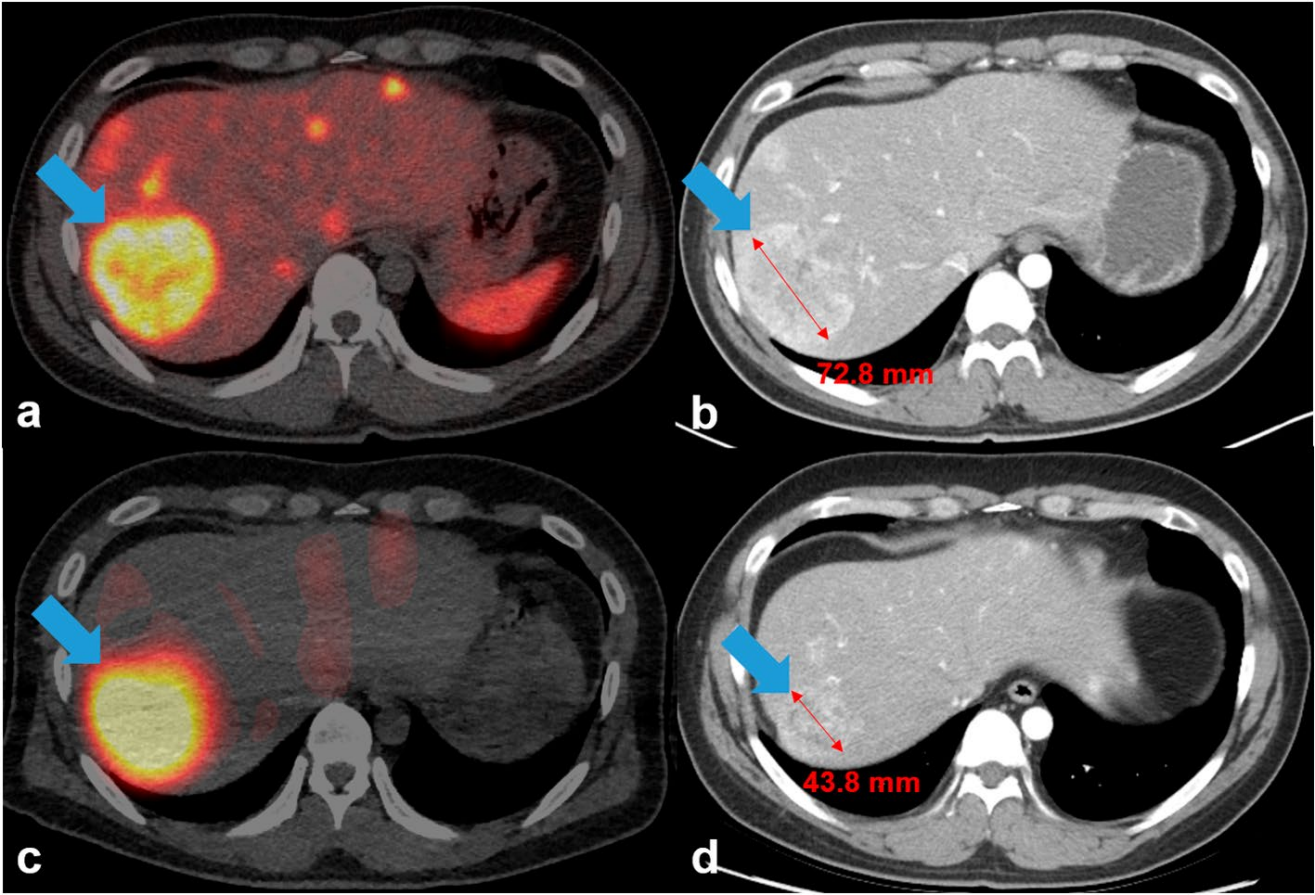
Representative images of the patient’s target lesion (liver) following [^177^Lu]Lu-DOTA-TATE therapy, showing a partial response (arrows). A Pre-therapeutic [^68^Ga]Ga-DOTA-TOC PET/CT exhibited a target lesion uptake in the liver that was more intense than normal liver uptake (Krenning score 3). B The lesion’s largest diameter measured 72.8 mm on pre-therapeutic CECT. After four cycles of [^177^Lu]Lu-DOTA-TATE therapy, C [^177^Lu]Lu-DOTA-TATE SPECT/CT, captured after the fourth PRRT cycle (SPECT/CT images after PRRT cycles 1–3 are not shown), revealed target lesion uptake in the liver that was more intense than normal liver uptake (Krenning score 3). The cumulative tumour-absorbed Dose_max_, Dose_peak_, and Dose_41_ of the target lesions were 157.8, 150.7, and 101.2 Gy, respectively. Following PRRT, the target lesion’s longest diameter decreased by > 30%, measuring 43.8 mm on (D) post-therapeutic CECT
